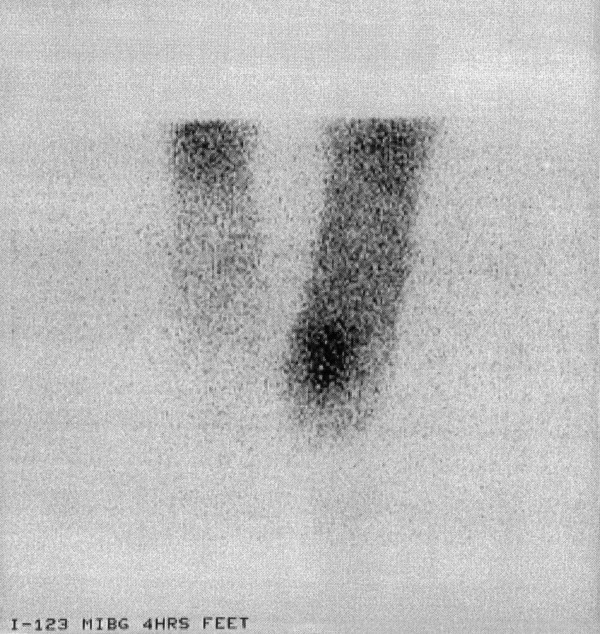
4 hour ^123^I mIBG scan of both feet in Case 2. There is increased uptake of radionucleotide corresponding to the lesion on MRI.
